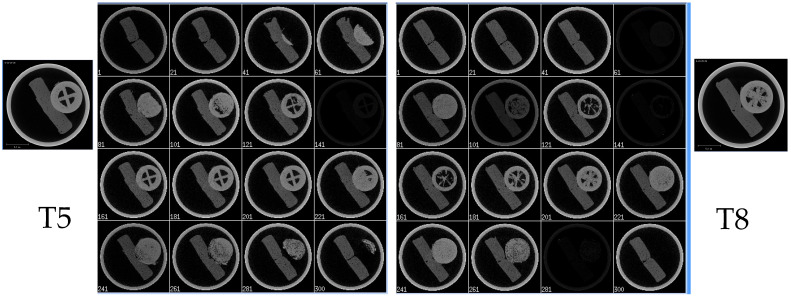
Axial CT slice for T5 and T8.
